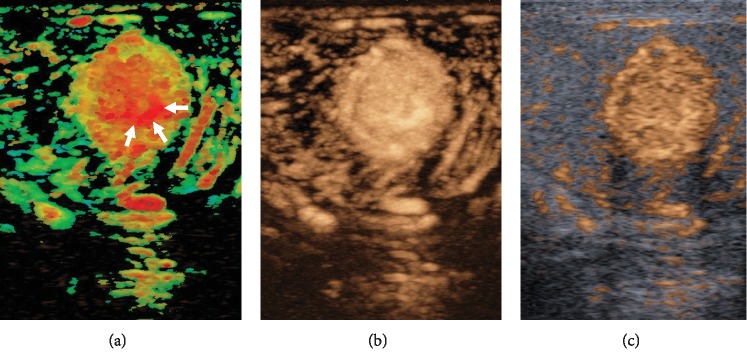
Arrival time imaging (ATI) and contrast-enhanced ultrasound (CEUS). (a) Parametric ATI depicted short arrival time in the central part of the tumor with feeder artery within 2 seconds (arrows; color-coded in red). (b, c) Accumulation mode and B-mode/CEUS image fusion demonstrates high peak intensity of the tumor compared to the surrounding testicular tissue. Abbreviations: ATI: arrival time imaging; CEUS: contrast-enhanced ultrasound.
